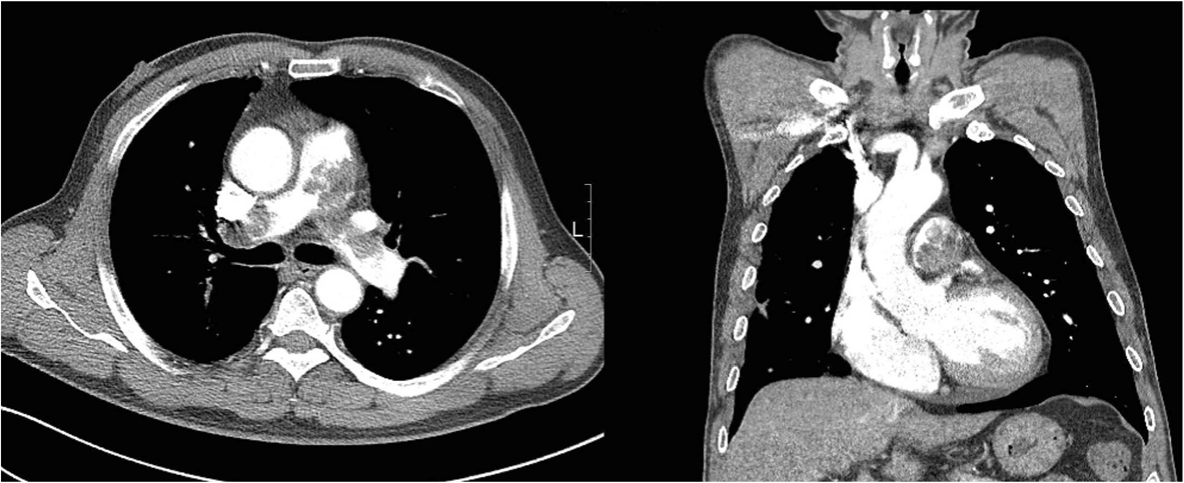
Chest computed tomography reveals multiple large masses in the main pulmonary artery and both branches. Main pulmonary artery and left pulmonary artery were almost completely blocked, and the right pulmonary trunk was completely blocked.
